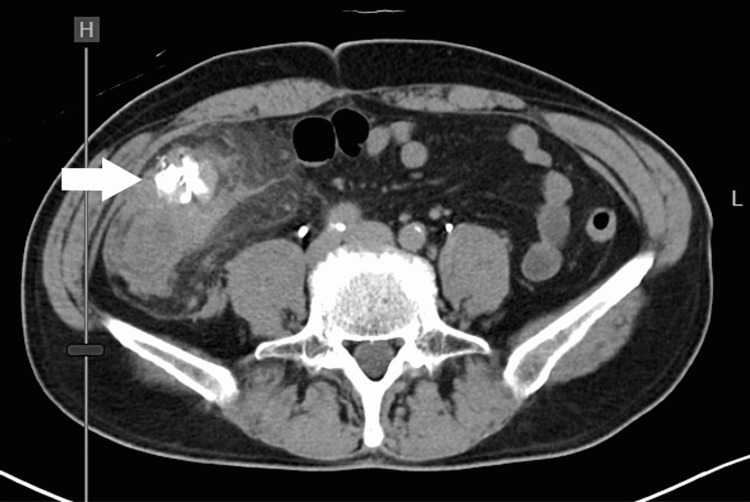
Abdominal plain CT scans, axial view CT scans revealed the presence of lipiodol in the pseudoaneurysm (arrow).
